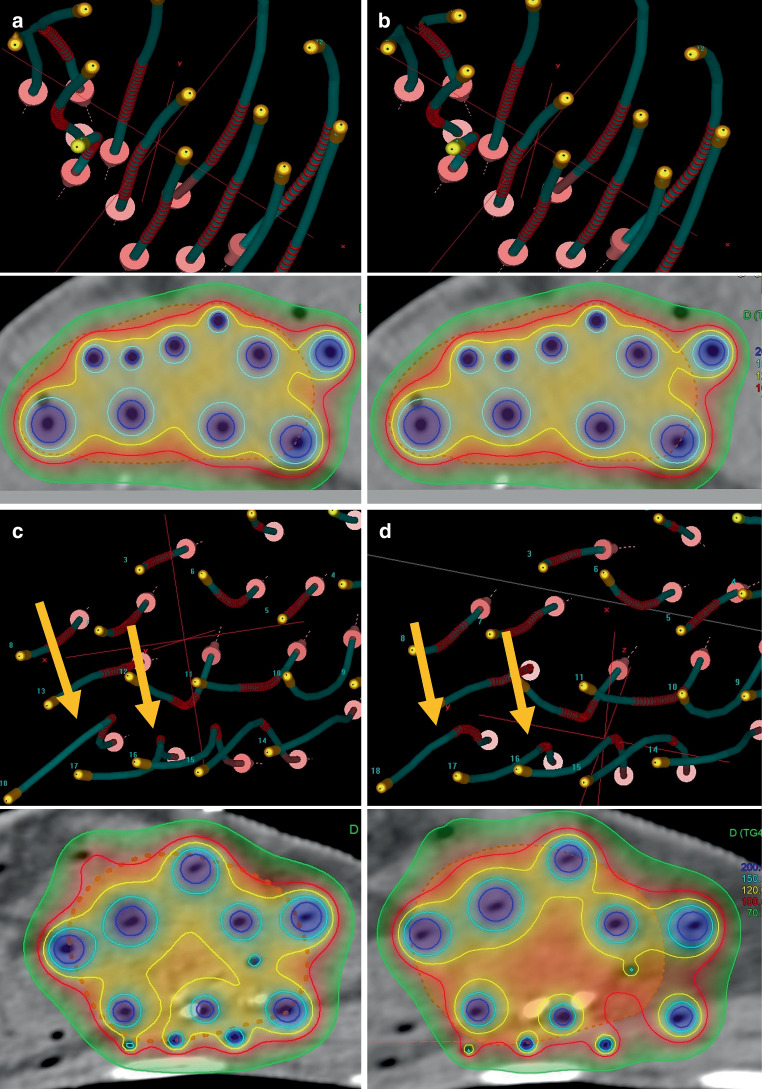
a and b show the planning-CT and control-CT situation, respectively, of a patient with very small geometric changes (dwell position deviations < 1 mm) treated with breast positioning control; c and d show the planning-CT and control-CT situation, respectively, of a previously treated patient with large dwell position deviations (up to 8 mm). In this case, catheters that clearly changed their paths within the breast are exemplarily marked with *orange arrows*. These changes resulted in an underdosage of some target volume parts. Catheter paths (*blue*) are in each case shown together with the source dwell positions (*red*) in the upper sections of each subfigure. The resulting dose distribution is visualized in the lower sections. The target volume is marked *orange* (*dashed*) in each case. The 70% (*green*), 100% (*red*), 120% (*yellow*), 150% (*cyan*), and 200% (*dark blue*) isodose lines are displayed
